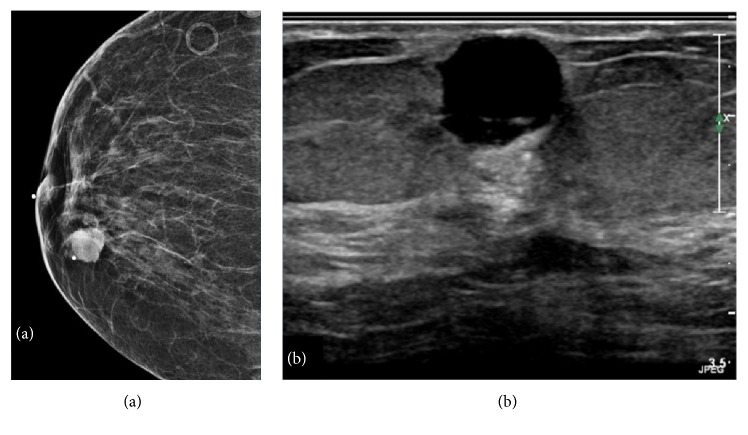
(a) Mammography, left breast: multinodular 19 mm predominantly solid lesion. (b) Ultrasonography, left breast: 19 x 13 x 12 mm complex solid and cystic lesion with internal vascularity located 2 cm from the nipple at 1:00 axis.
